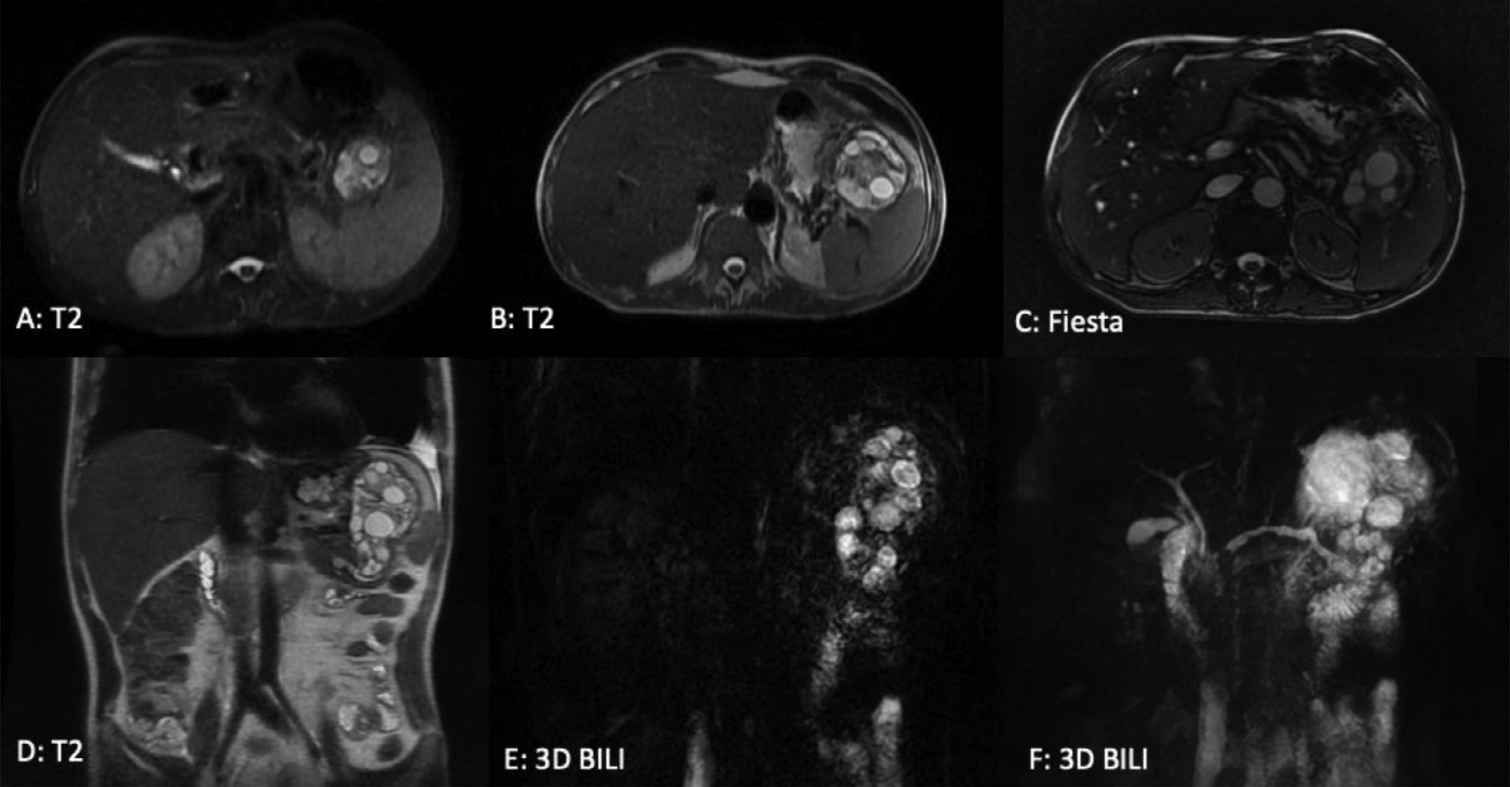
axial section (D-F) coronal section of a Bili-MRI showing a hydatid cyst causing a stage E pancreatitis.
